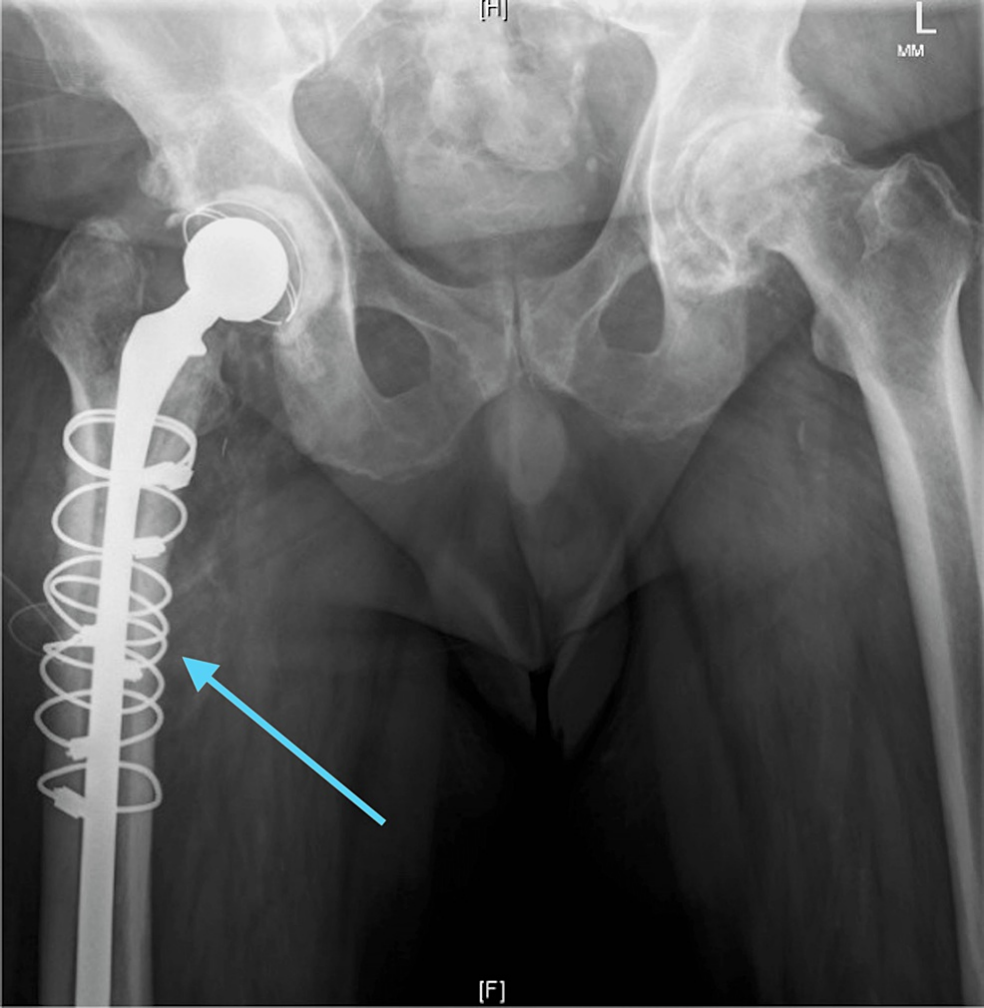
X-ray after explantation of the femoral and acetabular components was performed with placement of an antibiotic spacer.
